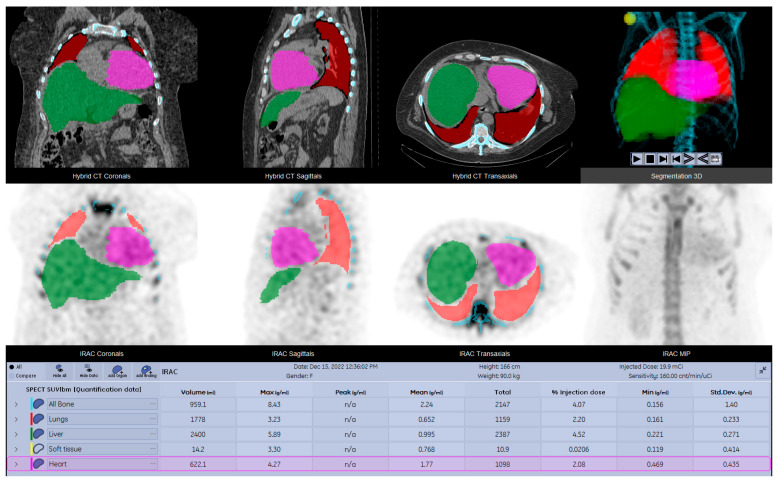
SPECT-CT data obtained with dedicated software; quantitative parameters for ratios, measurements, and objective image interpretation; bone, lungs, liver, soft tissue, and heart.
